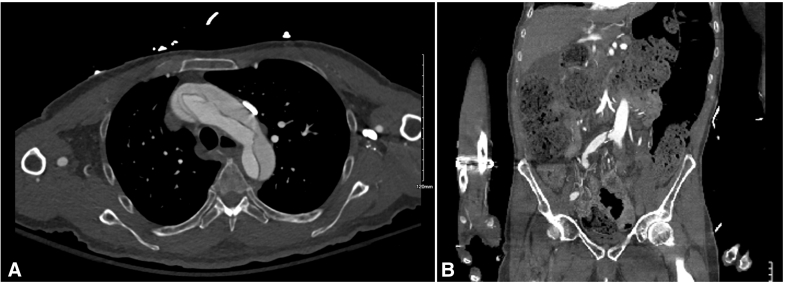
A, Stanford type A thoracic aortic dissection as viewed by computed tomography angiography. B, Left iliac occlusion secondary to the propagated dissection flap.
